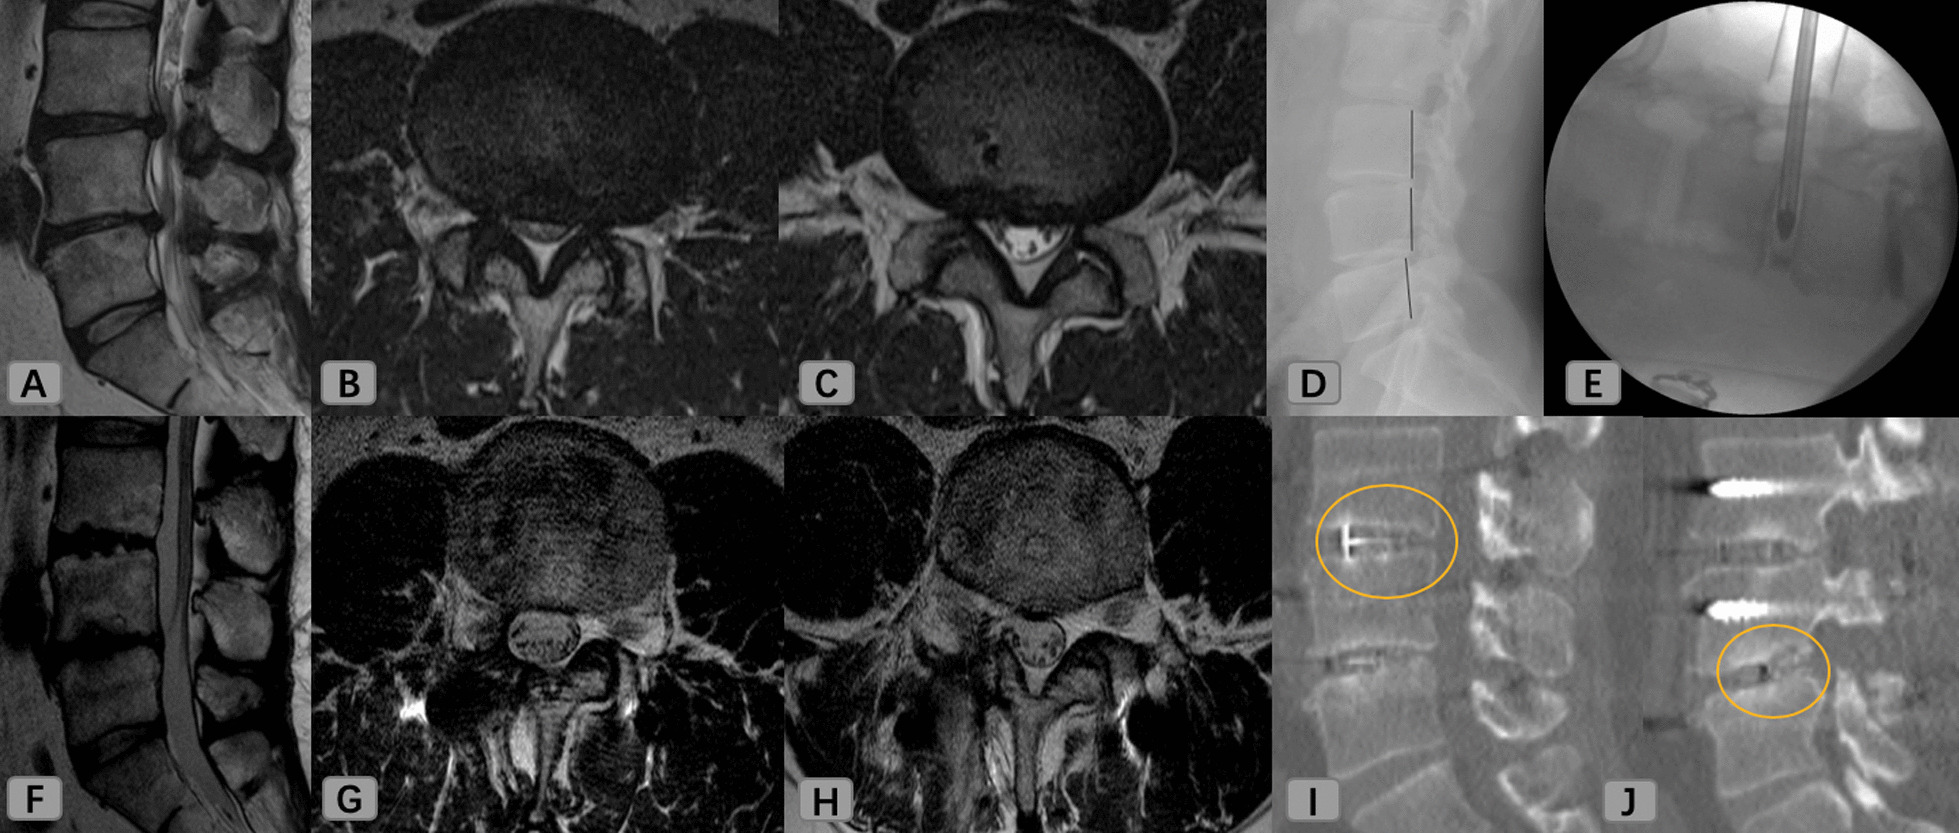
An age 49 male patient underwent endoscope-assisted minimally invasive transforaminal lumbar interbody fusion with fluoroscopy-guided technique at L3–4–5 level. A–C MR images show severe central stenosis at L3/4 (B) and L4/5 (C). D X-ray images show spondylolisthesis at L4/5. E An endoscope was introduced. F–H 1-year postoperative MR images. I, J Bony fusion (yellow circle) shows in coronal and sagittal computed tomography images at 6-month postoperatively
